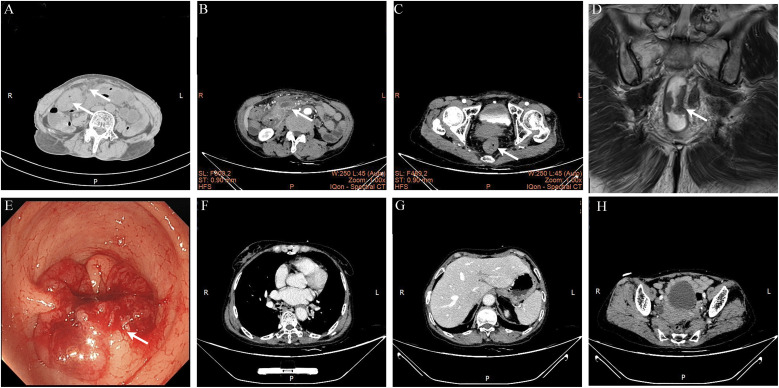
Imaging and colonoscopic findings. (A) Emergency non-contrast abdominopelvic CT, with arrows indicating the ascending colon mass and abdominal inflammatory changes; (B) Contrast-enhanced abdominopelvic CT, with an arrow indicating the ascending colon mass; (C) Contrast-enhanced abdominopelvic CT, with an arrow indicating the rectal mass; (D) T2-weighted high-resolution pelvic MRI showing the rectal mass; (E) Colonoscopy image, with an arrow indicating the severe intestinal stenosis caused by the rectal mass; (F-H) Surveillance thoracoabdominal CT at 24-month follow-up, showing no evidence of tumor recurrence or metastasis.
